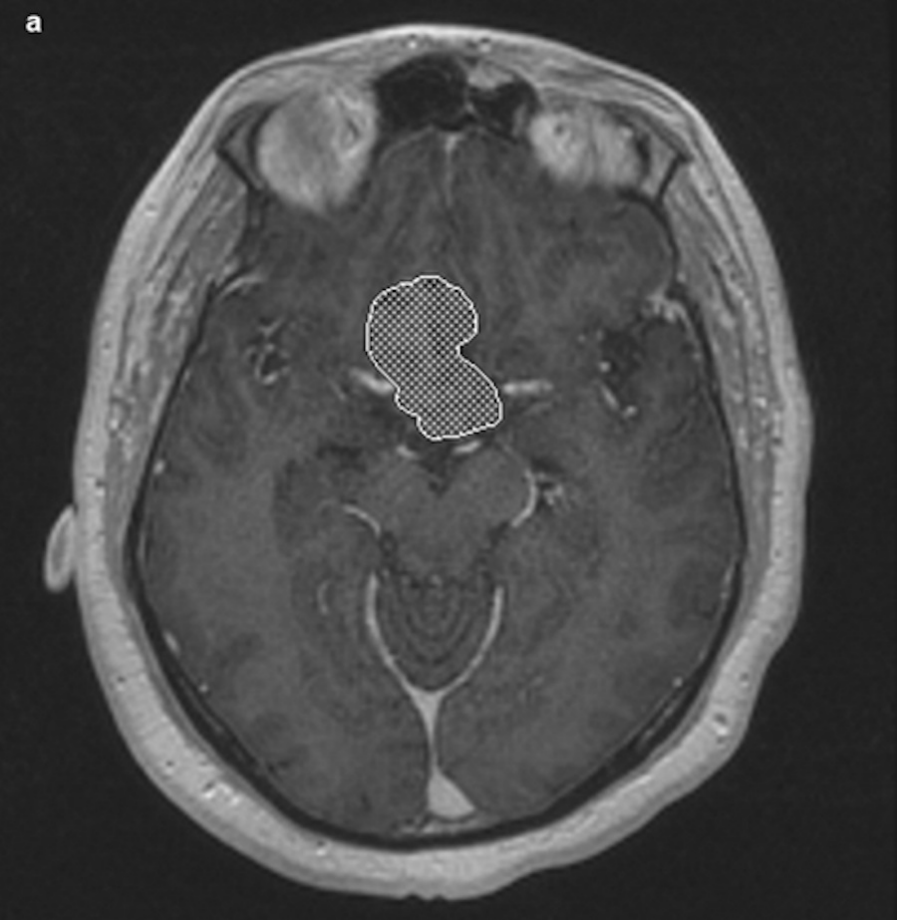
Representative axial spoiled gradient recall echo (SPGR) MR image of preoperative pituitary tumor post-volumetric analysis highlighting tumor silhouette
